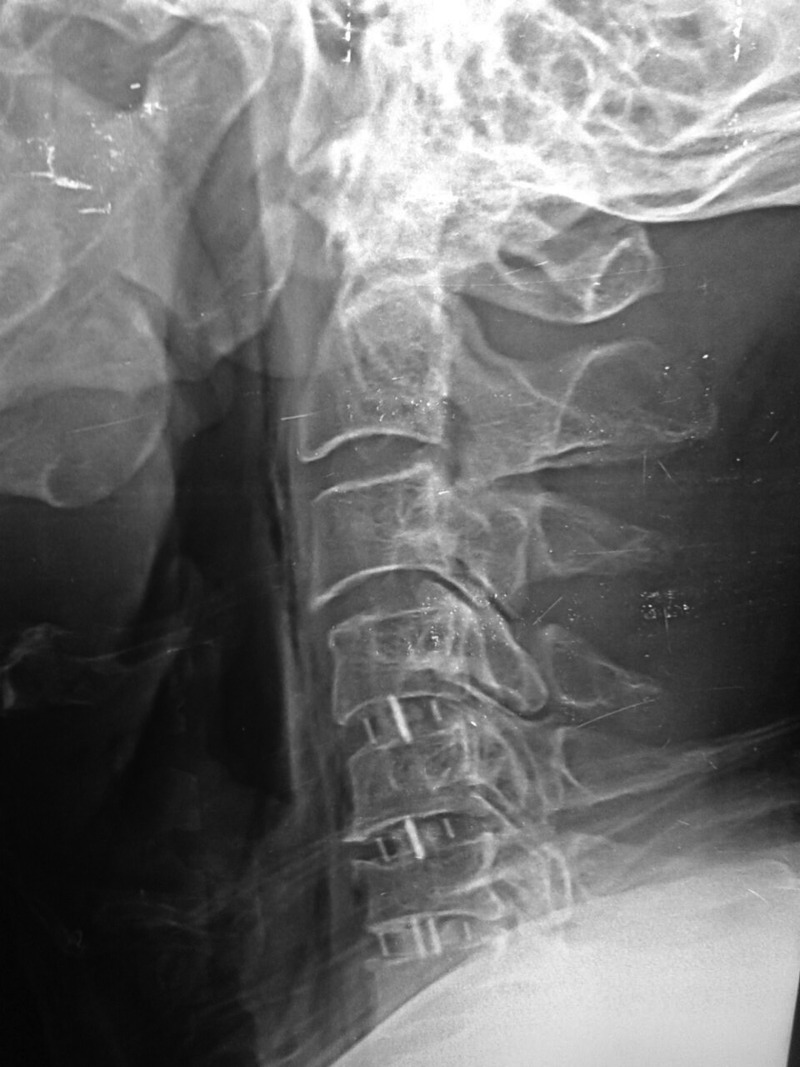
Case Example of a Three-Level ACDF Using Stand-Alone PEEK Cages Sagittal radiograph at three months after surgery demonstrating hardware placement and preservation of disc space heights.
